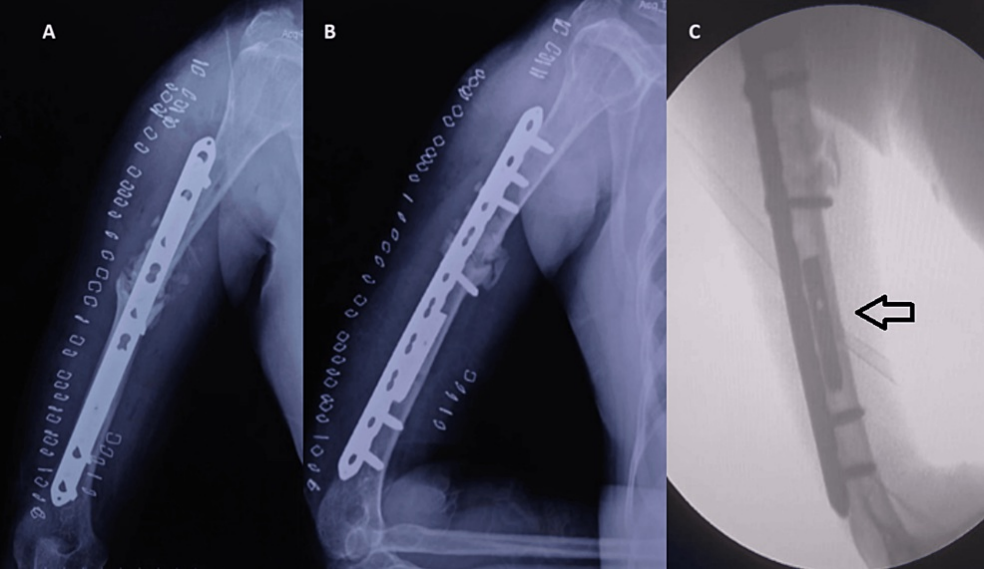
Postoperative radiograph of failure of Intramedullary nailing managed with dynamic compression plating and bone grafting (A) Lateral view; (B) anteroposterior view; (C) fluoroscopic intraoperative image with an arrow showing a retained piece of Intramedullary nail within the humeral shaft canal
